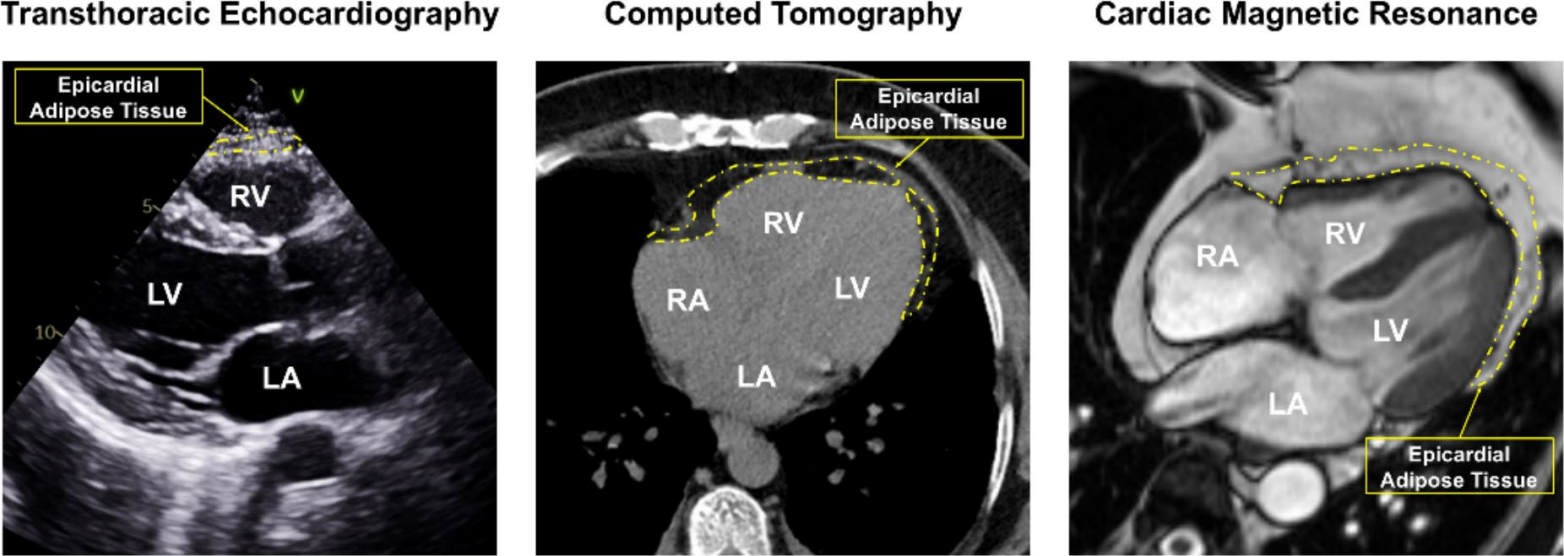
Cardiovascular imaging techniques for measuring epicardial adipose tissue thickness and volume. Epicardial adipose tissue (EAT) can be measured as thickness using transthoracic echocardiography (left), or as single-slice areas or multi-slice volumes using computed tomography (middle) and cardiac magnetic resonance (right) imaging techniques. EAT is displayed within yellow borders. *Abbreviations: RA, right atrium; RV, right ventricle; LA, left atrium; LV, left ventricle*
